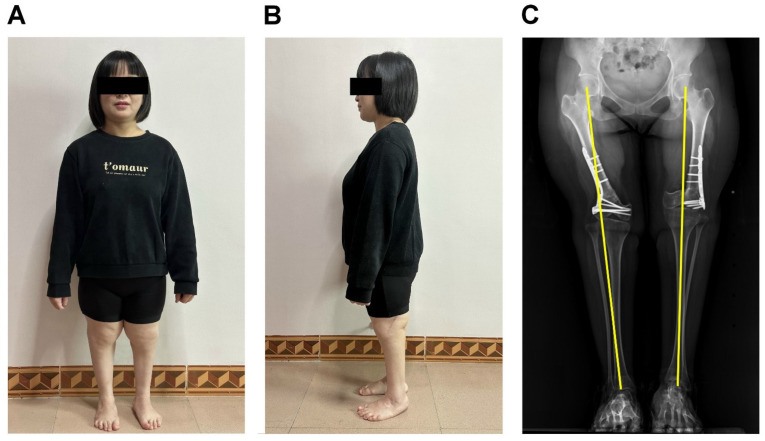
Postoperative 9-year photograph and radiography of the patient in the standing position. The front (A) and lateral photograph (B) show normal standing posture. The anterior–posterior triple film shows good alignment of the mechanical axis (yellow line) (C).
